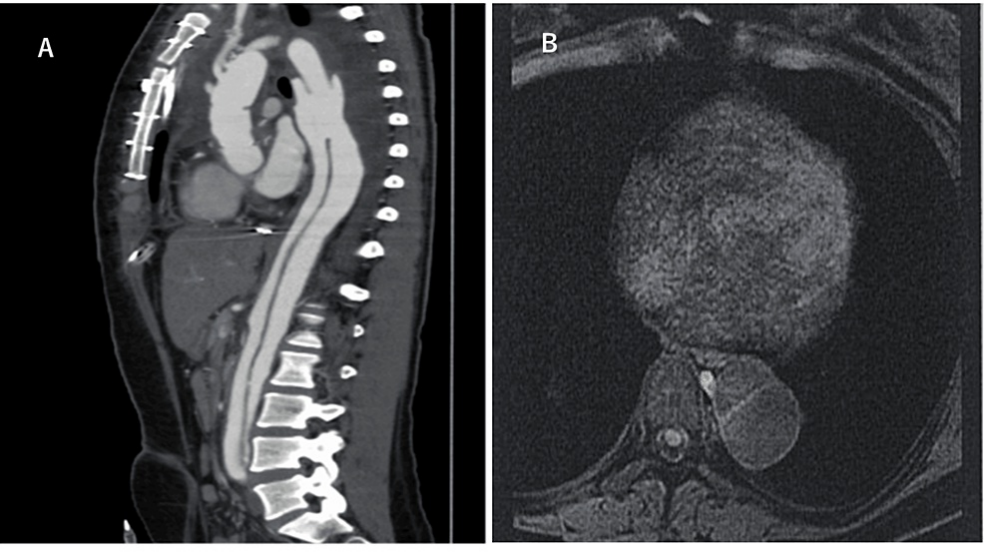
Contrast computed tomography image and MRI T2-weighted image A: Contrast computed tomography image taken before pregnancy shows residual aortic dissection from the distal arch aorta to just above the common iliac artery bifurcation. B: MRI T2-weighted image taken at 37 weeks' gestation shows a residual dissected cavity in the distal arch of the replacement, but no diameter enlargement.
